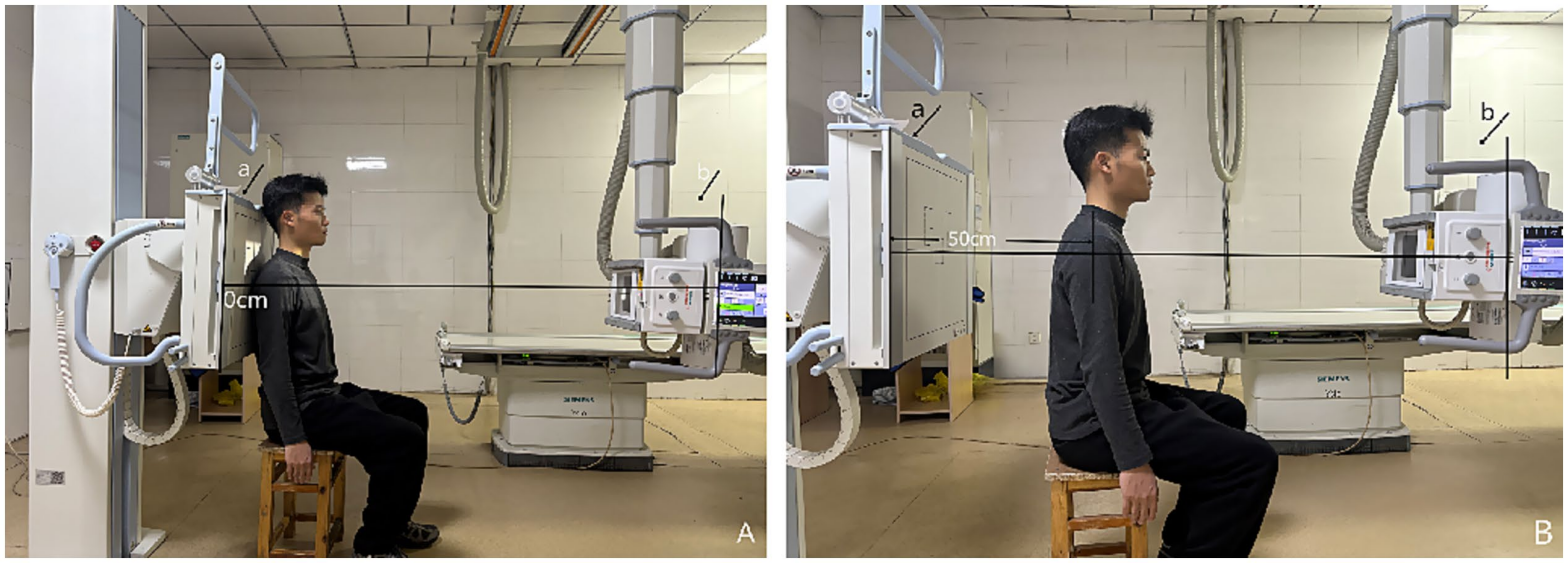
Standard position for radiography of the shoulder joint. (A) The distance from individuals to the flat panel detector is 0 cm. (B) The distance from individuals to the flat panel detector is 50 cm. a—The flat panel detector, b—The tube.
